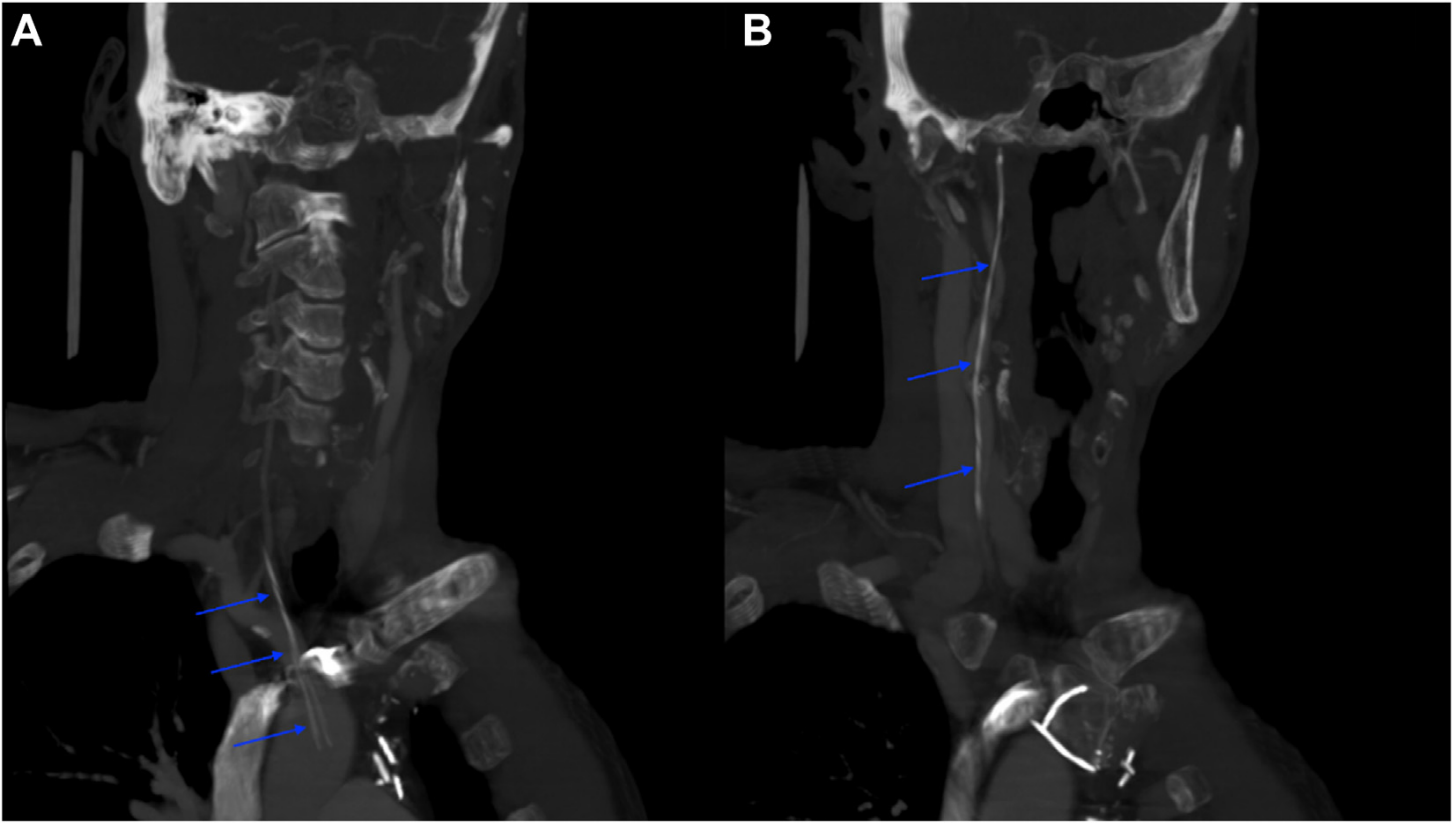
CT Neck Demonstrating the TEPW Migration Into Carotid Artery (A) Computed tomography of the neck with intravenous contrast showing the retained temporary epicardial pacing wire (TEPW) coursing through the brachiocephalic artery into the right common carotid artery, indicated by the blue arrows. (B) The same scan shows the retained TEPW extending from the right common artery, as indicated by the blue arrows.
